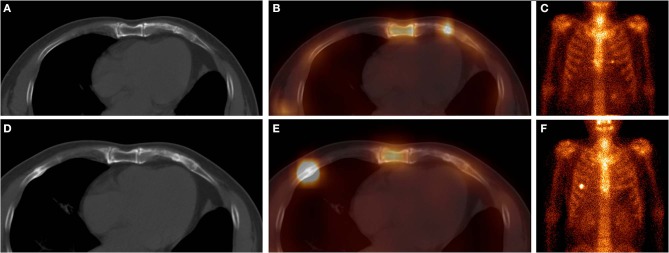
These images were obtained from a 65-year-old man with atraumatic CCF in the SG, who underwent chemotherapy and surgery for left lung adenocarcinoma 3 months ago. ^99m^Tc-MDP SPECT/CT axial images (A, CT; B, fusion) and WBS image (C, anterior) demonstrated a clear fracture line in the calcification of the left fifth costal cartilage, with high-uptake tracer activity. After 9 months, the SPECT/CT images (D, CT; E, fusion) and WBS image (F) showed disappearance of the fracture line of the left fifth costal cartilage, local callus formation, and normal uptake. Moreover, a new focus of intense tracer was noted in the right fifth anterior rib, with callus formation. These indicated that this patient was at a high risk of fracture.
